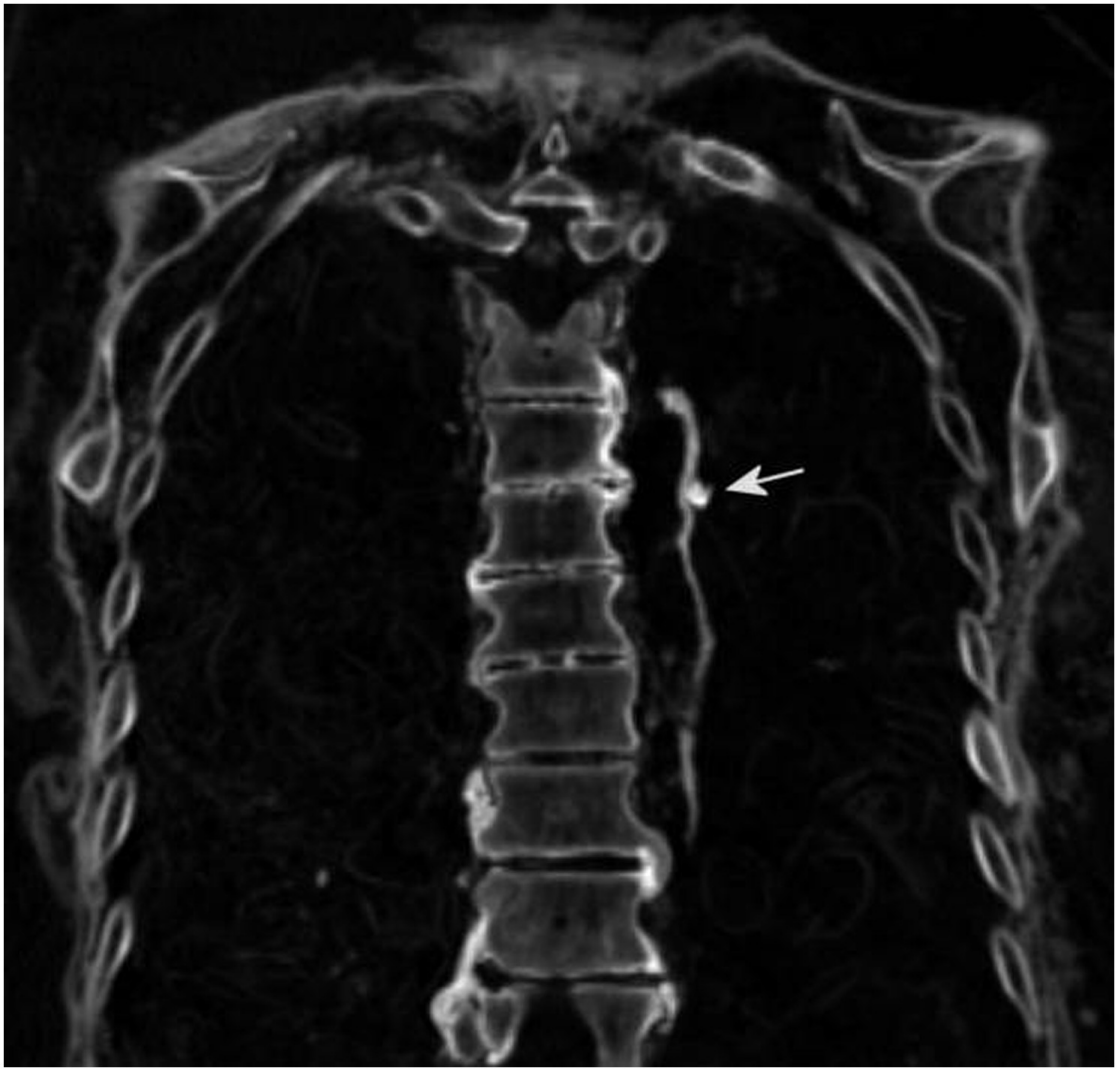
Sample of the CT scans of the lungs: Here the retracted left lung shows hilar calcifications (arrow) and significant osteophytosis of the vertebrae.
